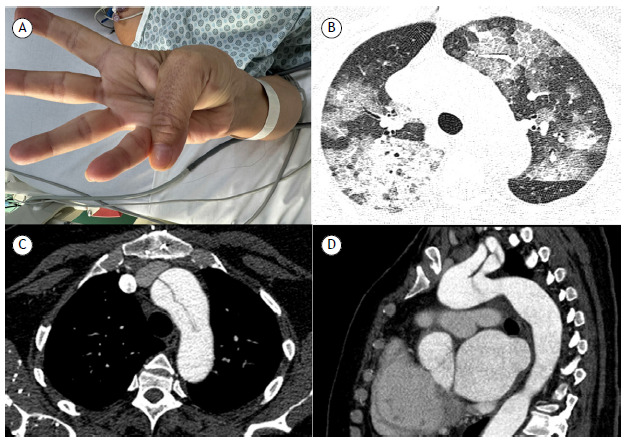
In A, clinical photograph showing hyperextensibility of the thumb. Note that the distal phalanx of the thumb extends beyond the ulnar border of the hand. In B, axial chest CT image (lung window) showing bilateral areas of ground-glass attenuation and interlobular septal thickening (a crazy-paving pattern). In C and D, respectively, axial and sagittal reconstructions (mediastinal window) showing areas of aortic dilatation and aortic stenosis, particularly in the ascending portion, as well as aortic dissection.
